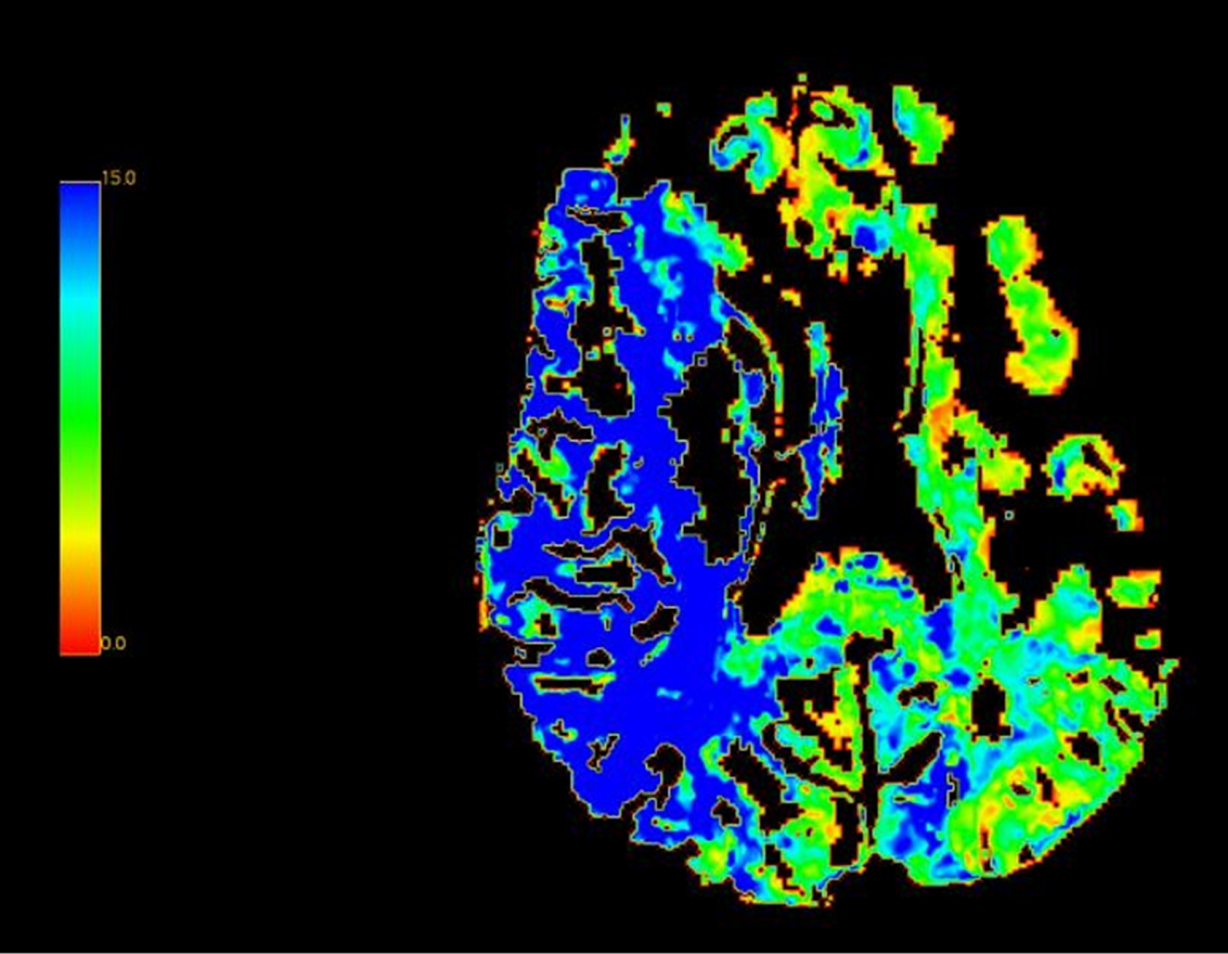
Computed Tomography Perfusion Study A computed tomography perfusion study shows decreased cerebral blood flow within the penumbra territory (right middle cerebral artery), represented by the blue coloration. Otherwise, normal perfusion is indicated by the green coloration.
